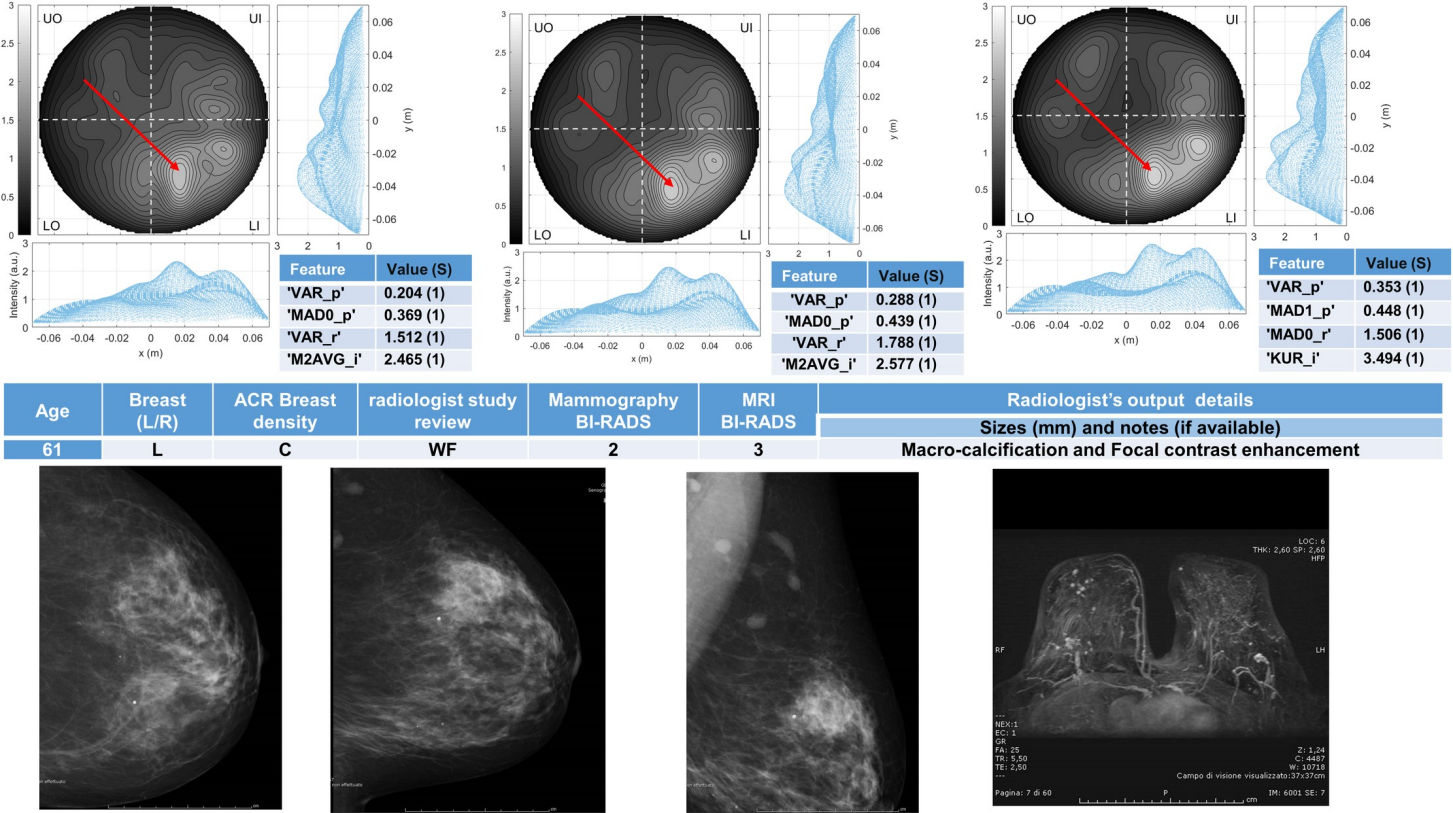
The radiologist study review “WF” for this heterogeneously dense (ACR C) breast has been obtained with the support of mammography and MRI images given in the bottom row, giving as output the presence of a macro-calcification and a focal contrast enhancement. The Mammography BI-RADS is 2 and the Magnetic Resonance Imaging (MRI) BI-RADS is 3. The final assessment is not available. Microwave images, normalized to unitary average of the intensity, are given in the top row for three different conductivity weighting (from left to right: 0.3 S/m, 0.4 S/m and 0.5 S/m, respectively). X and Y are given in meters; intensity is in arbitrary units. All microwave images show a non-homogeneous behavior, with a main peak indicated by the red arrows; a slightly lower peak in the same quadrant may be also noted. The binary score S (given in the inserted tables) is 1 for the all the features. The proposed *rule-of-thumb* classifies this breast as positive.
